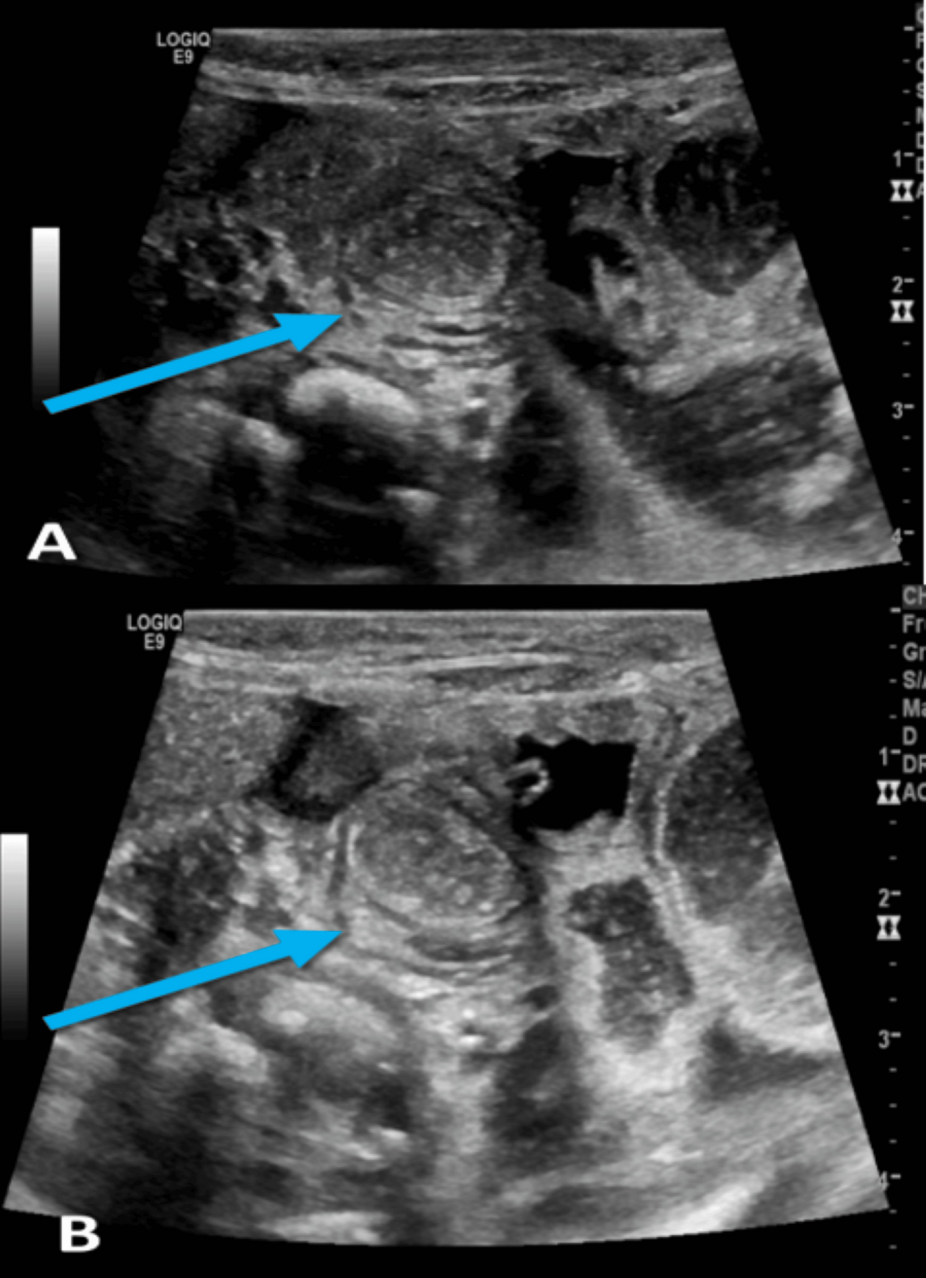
Ultrasound abdomen: A. Cylindrical mass with an outer hypoechoic ring (blue arrow). B. Showing the appearance of a target sign (blue arrow)
